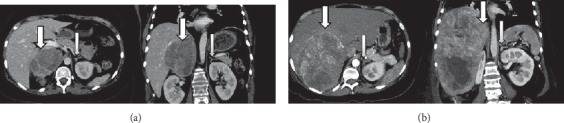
High-resolution, contrast abdominal CT scan, at diagnosis (a) and 16 months after right adrenalectomy (b). (a) Axial and coronal views showing a large, heterogeneous right adrenal mass and an atrophic left adrenal gland. (b)Axial and coronal views showing a large, heterogeneous hepatic mass and a somewhat hypoplastic left adrenal gland.
